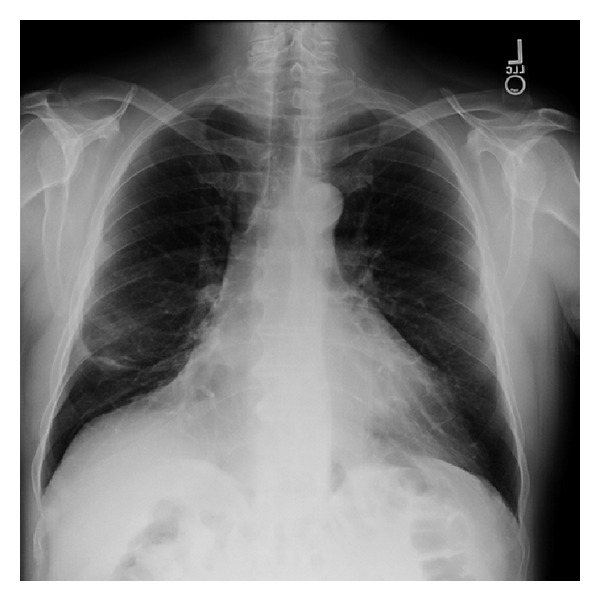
Chest radiograph demonstrating near-complete resolution of the right middle lobe mass after treatment with corticosteroids.
